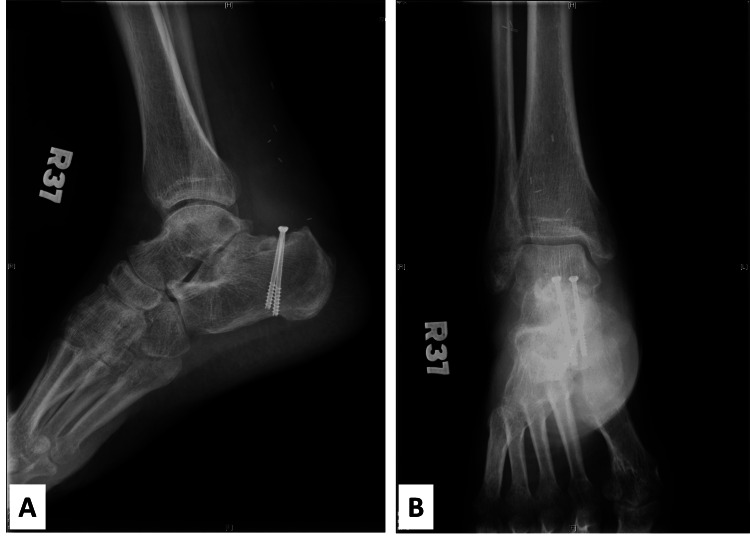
X-ray images to the recipient site X-ray images depicting adequate bone union between the recipient site and a bony portion of the allograft. (A) Lateral view. (B) Anteroposterior view.
